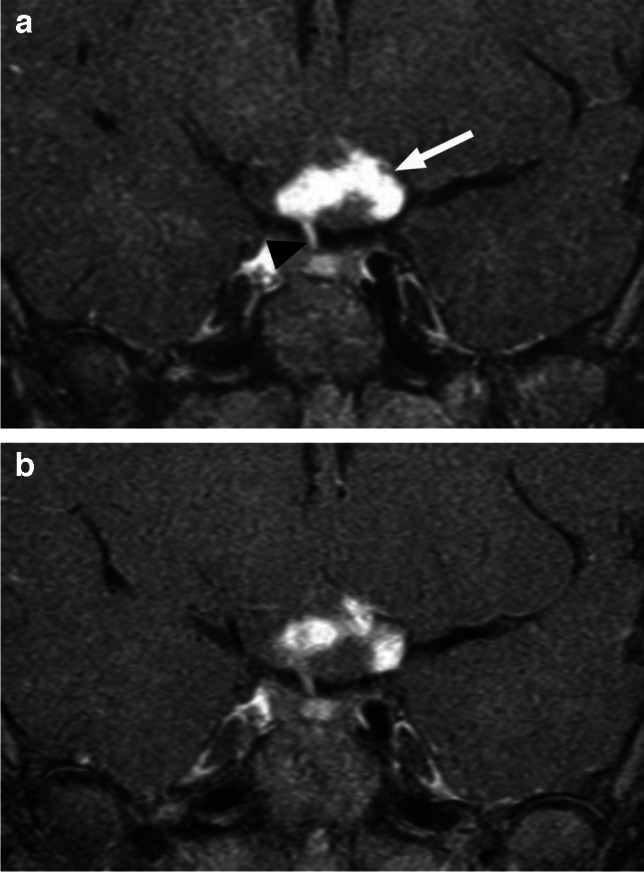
OPG in a patient with NF1. Coronal T1-weighted postcontrast image with fat saturation (a) shows an optic chiasm/hypothalamic mass with avid heterogenous enhancement (arrow), adjacent to pituitary infundibulum (arrowhead). Corresponding follow-up image (b) reveals decreased and more heterogenous enhancement, without any interval treatment
